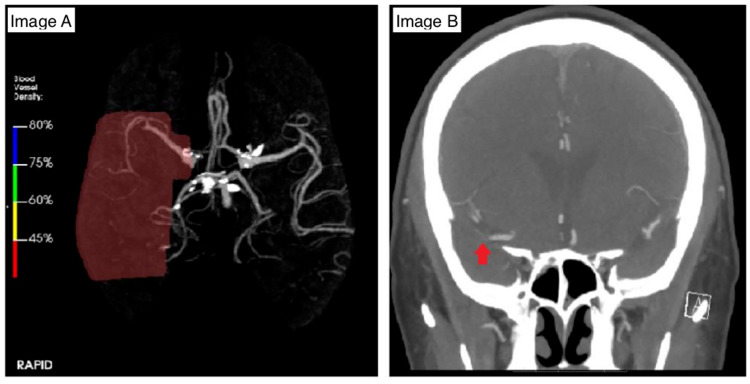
Computed tomography angiography of the patient's brain. Image A: diminished blood vessel density in the right middle cerebral artery (MCA) distribution (highlighted red area) with low blood vessel density due to occlusion of the major M2 and M3 branches; Image B: right-sided M2 branch occlusion (red arrow).
